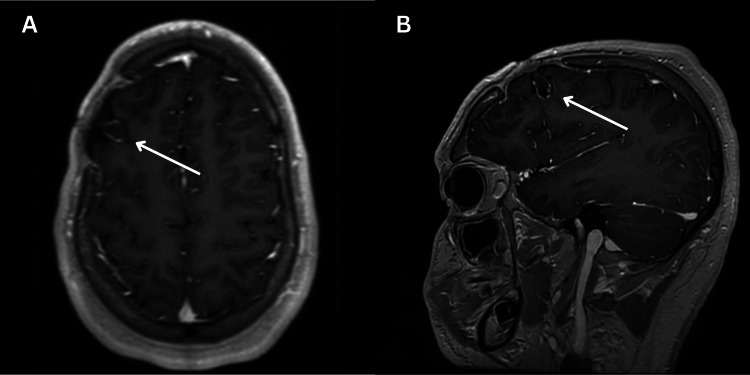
Head imaging at the eight-month follow-up Axial (A) and sagittal (B) view of a post-gadolinium T1-weighted brain MRI at the eight-month follow-up showing a small (9.3 x 8.6 mm) rim-enhancing collection within the right frontal lobe.
